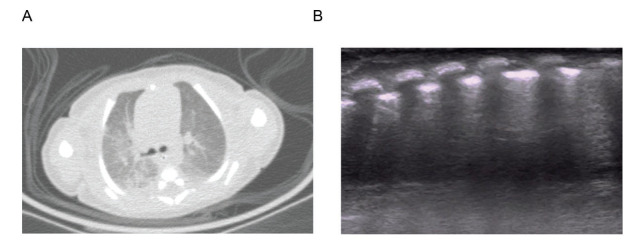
Baby ACO pulmonary imaging exam (case 2). A. Chest computed tomography: Opacities and atelectasis band in the right upper lobe. Slightly diffuse increase in attenuation of the lung parenchyma. B. Left chest ultrasonography: Presence of coalescent B lines and sub-pleural consolidations on the left posterior base.
